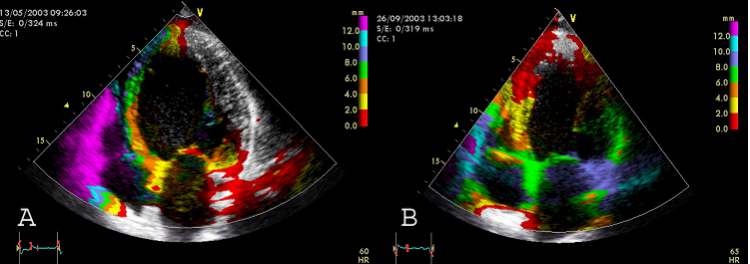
Demonstration of successful BVP by Tissue Tracking in apical four chamber view in a patient with dilated cardiomyopathy. Images from apical four chamber view. A) Before BVP, there is a dilated ventricle ("baseball shape") with reduced systolic displacement (max displacement = 8 mm) in the septum and DLC in the lateral wall (no colour-coding) indicating asynchrony of the lateral wall. B) After three months of BVP, there is a reduction of left ventricular dilatation (reverse remodelling, "American football shape" of the left ventricle), increased tracking values and no DLC regions anymore.
